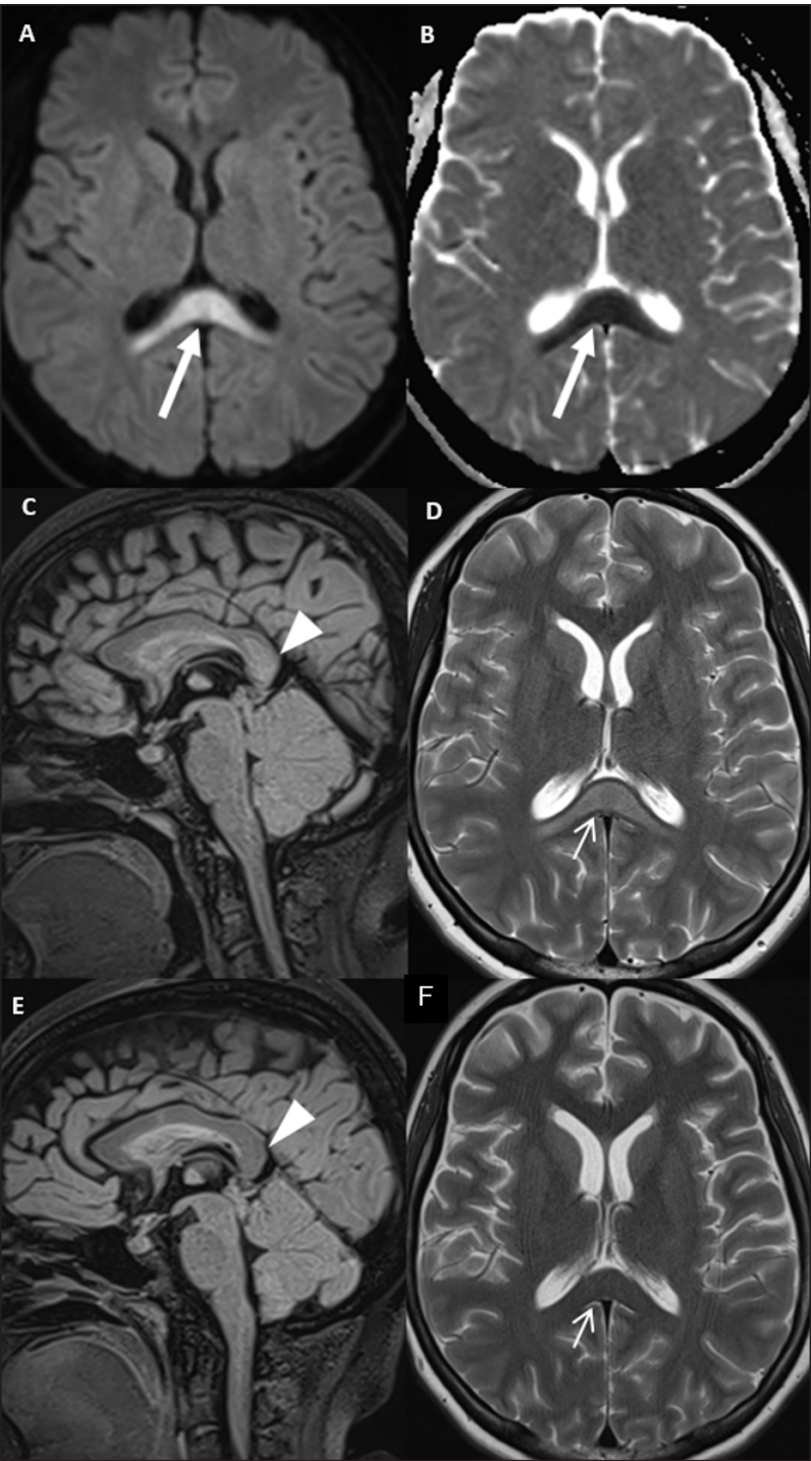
Axial DWI and ADC images A and B showed diffusion restriction in the splenium of the CC (long arrows showing high signal intensity on DWI on image A and low signal intensity on ADC on image B) with a high signal intensity on the sagittal FLAIR on image C (arrowhead showing high signal intensity in the splenium) and a high signal intensity on the axial T2 on image D (short arrow showing high signal intensity in the splenium) suggesting a CLOCCS. Sagittal FLAIR on image E (arrowhead showing the disappearance of the high signal intensity in the splenium) and axial T2 on image F (short arrow showing the disappearance of the high signal intensity in the splenium) showed a complete resolution of the CLOCCS signs one week later.
